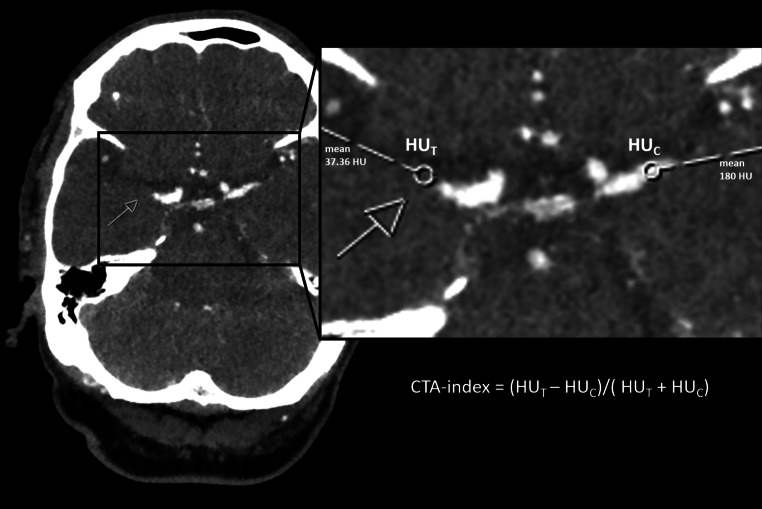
Example of CTA-index assessment for a patient that underwent admission CTA imaging of an acute occlusion of the right middle cerebral artery within the M1 segment. The ROIs for measurements of mean HU were placed 1.5 mm behind the occlusion site (T) and at a corresponding position of the contralateral, not occluded vessel (C)
